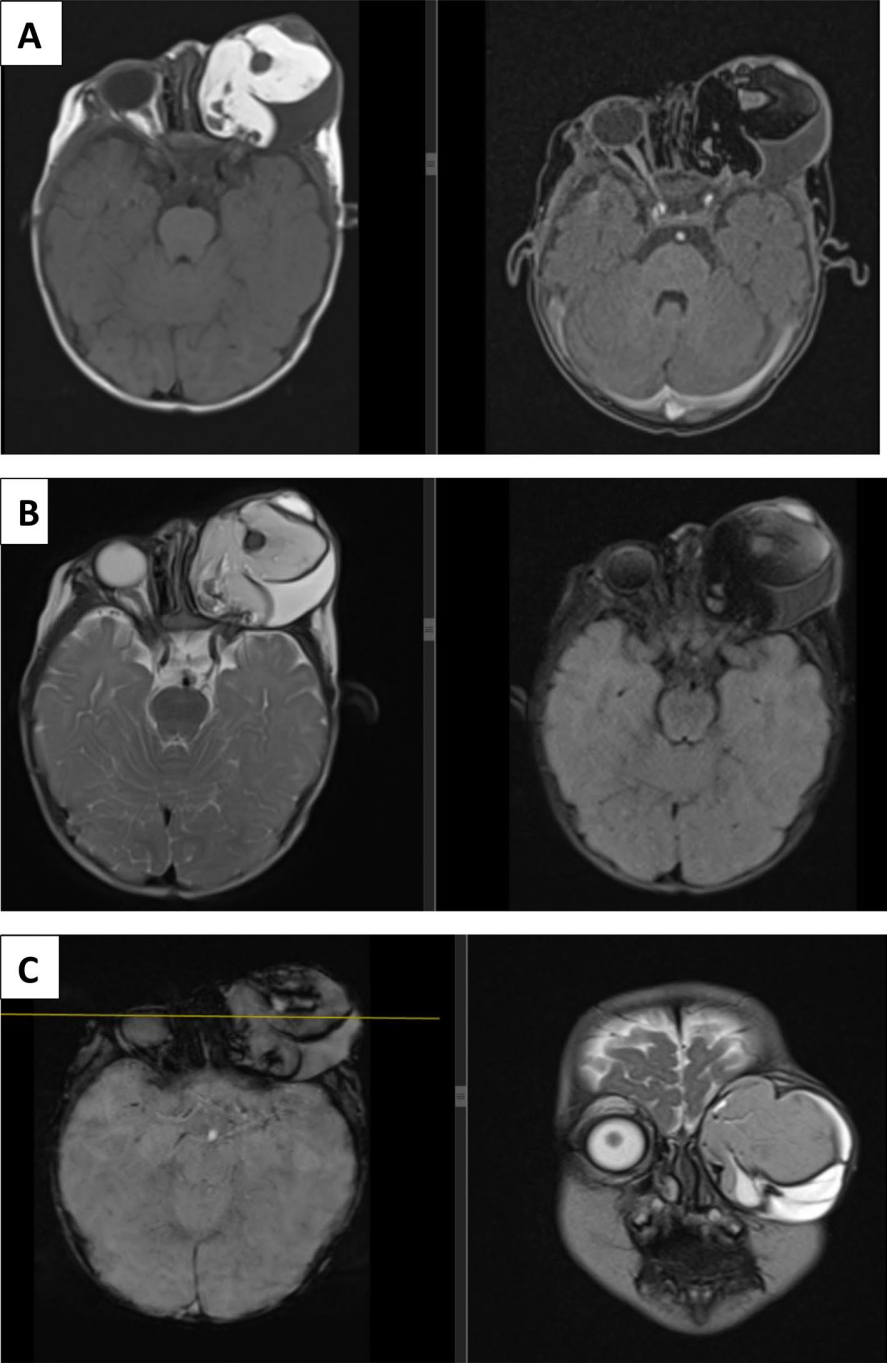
(A) T1 and T1 FS axial images showing heterogenous mass with a fat component in left orbital cavity. Septal smooth enhancement was observed. (B) T2 and FLAIR FS images showing heterogenous mass in left orbital cavity with cystic and fat component. (C) SWI and T2 coronal images showing the left orbital mass with blooming regions
